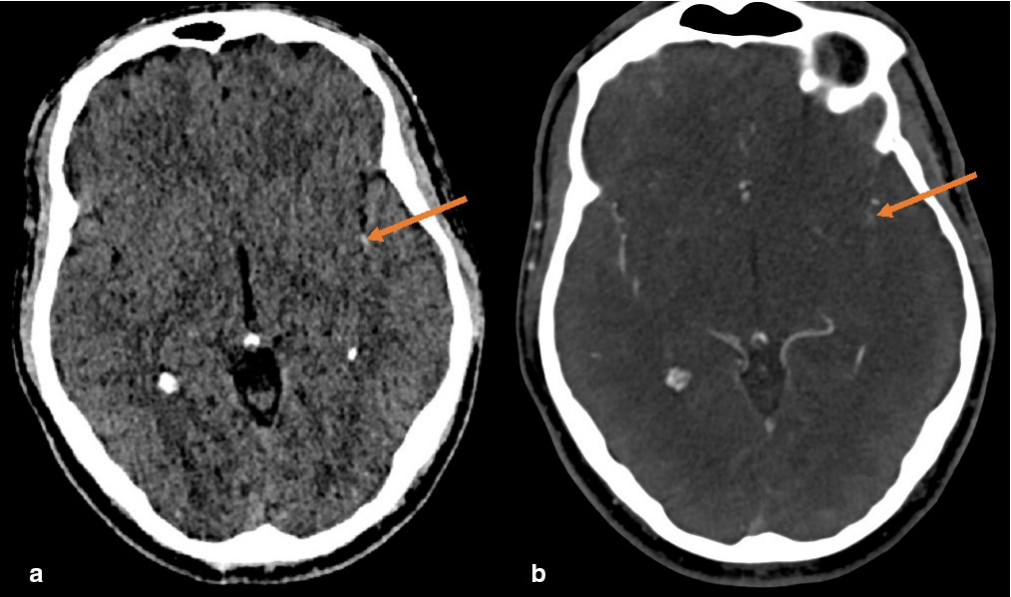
Unenhanced CT brain demonstrating hyperdense insular segment of left MCA representing hyperacute thromboembolic material within the vessel lumen (a). Corresponding axial intracranial CT angiogram demonstrating a proximal occlusion of the left posterior M2 MCA division (b). MCA, middle cerebral artery.
